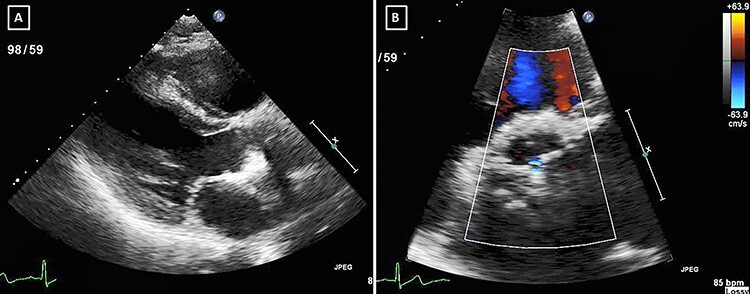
Initial TTE showing no evidence of para-aortic collection (A) parasternal long-axis view, (B) short-axis view with colour Doppler.
